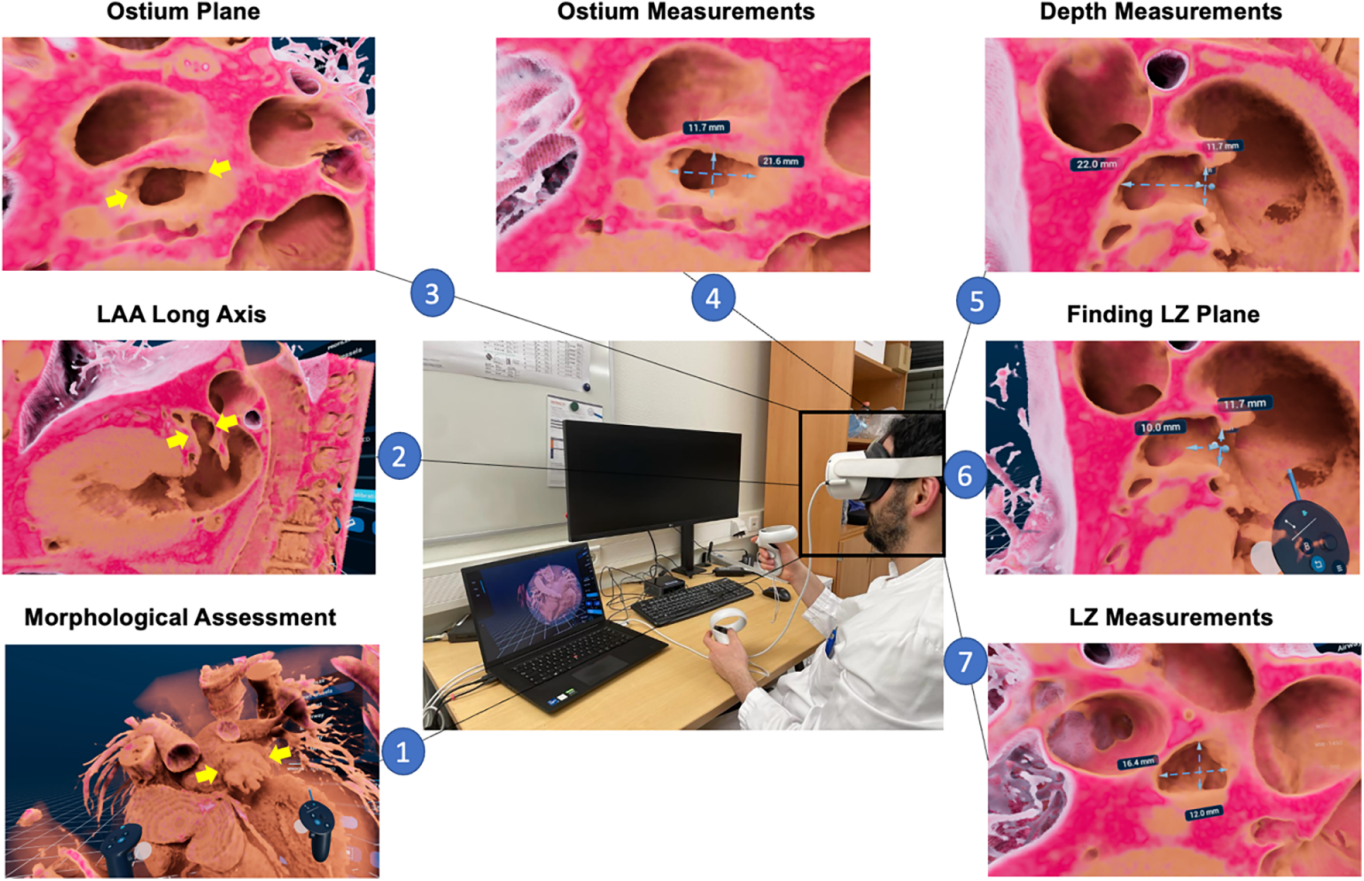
LAA sizing protocol in VR. First, an overview of 3D rendered patent-specific MSCT dataset is displayed in VR environment and the LAA morphology (yellow arrows) can be assessed (1). Next, by cutting through the LAA and the long axis of the left ventricle, the long axis of the LAA is displayed and can visually be assessed (2); subsequently, by cutting through the left atrium perpendicular to the ostium, an en-face view of the LAA orifice is achieved (3), and minimum and maximum diameters can be measured (4); then, the depth from the ostium to LAA roof is measured (5) and at a distance of 10-12 mm distal from the ostium (6) the landing zone minimum and maximum diameters are measured (7); MSCT, multi-slice computed tomography; VR, virtual reality; LAA, left atrial appendage; LZ, landing zone.
